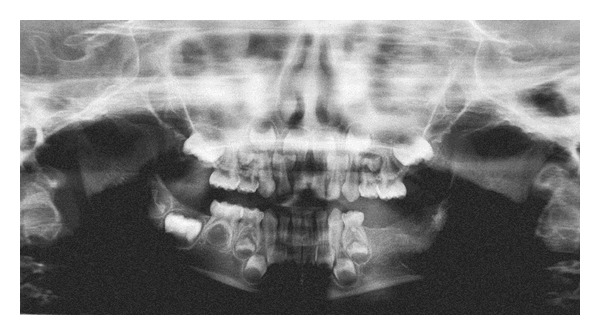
Postoperative panoramic radiograph, demonstrating that the radiodensity of the lesion area is similar to normal bone, indicating complete bone regeneration.
